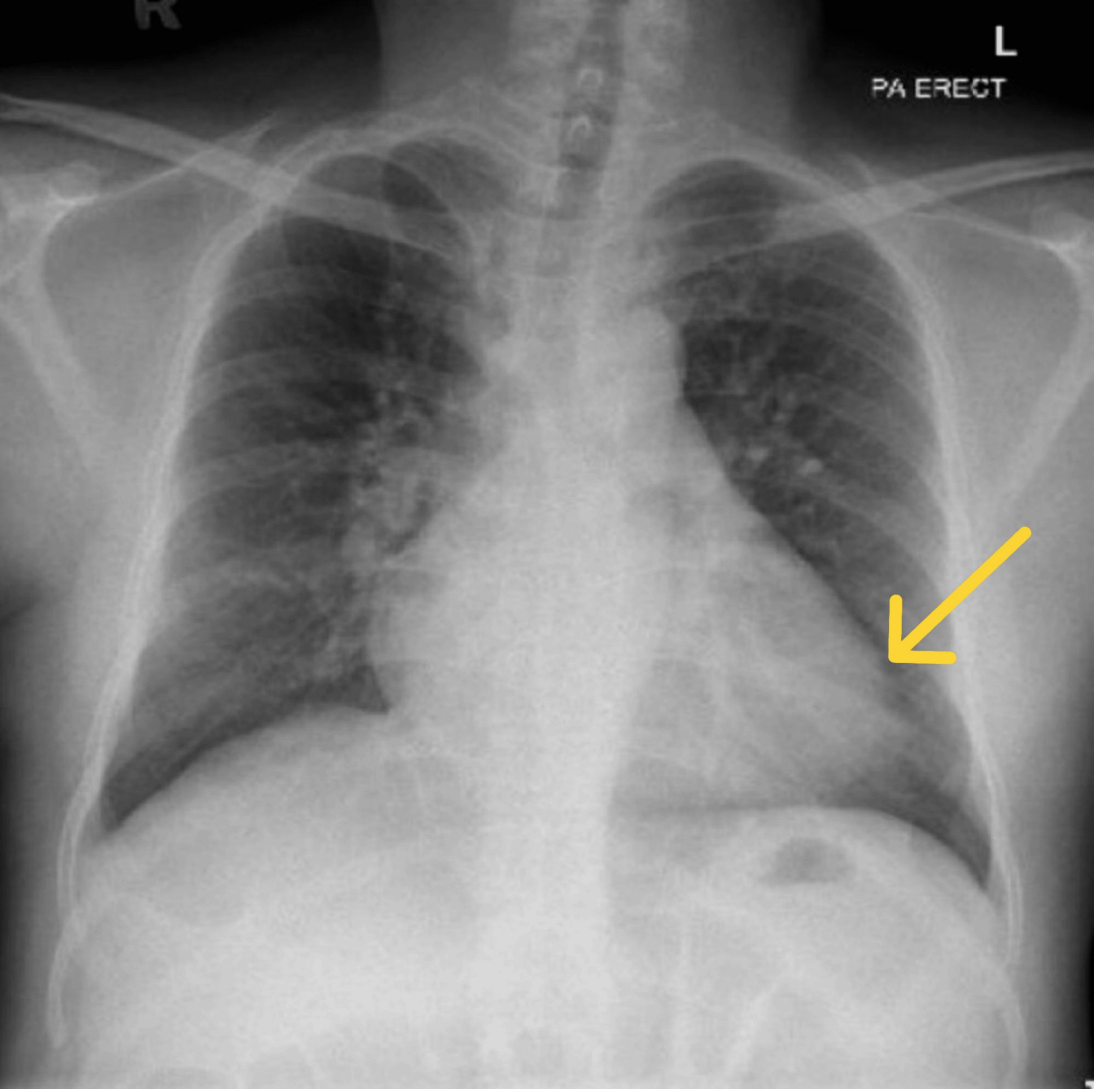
A chest X-ray posteroanterior view revealed mild cardiomegaly.
